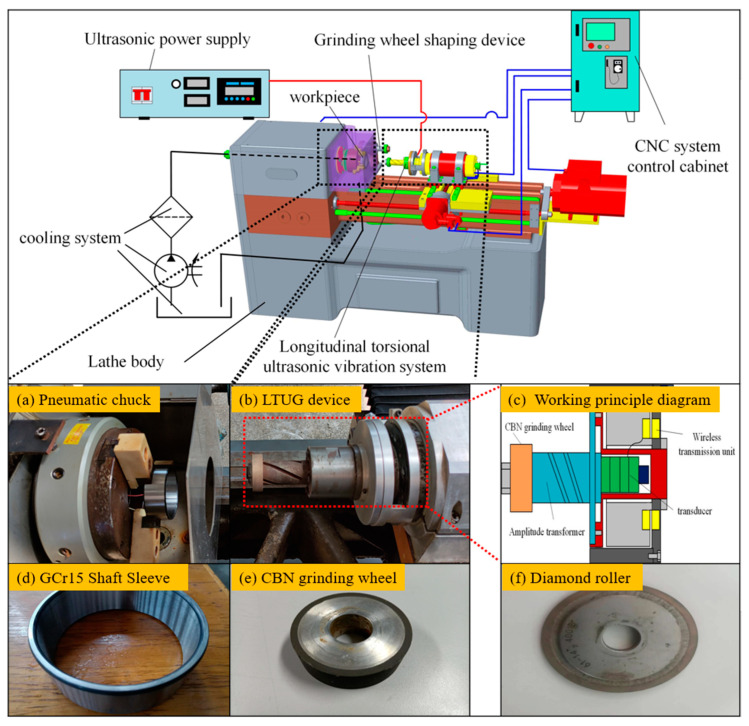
CNC longitudinal torsional ultrasonic internal grinder.
